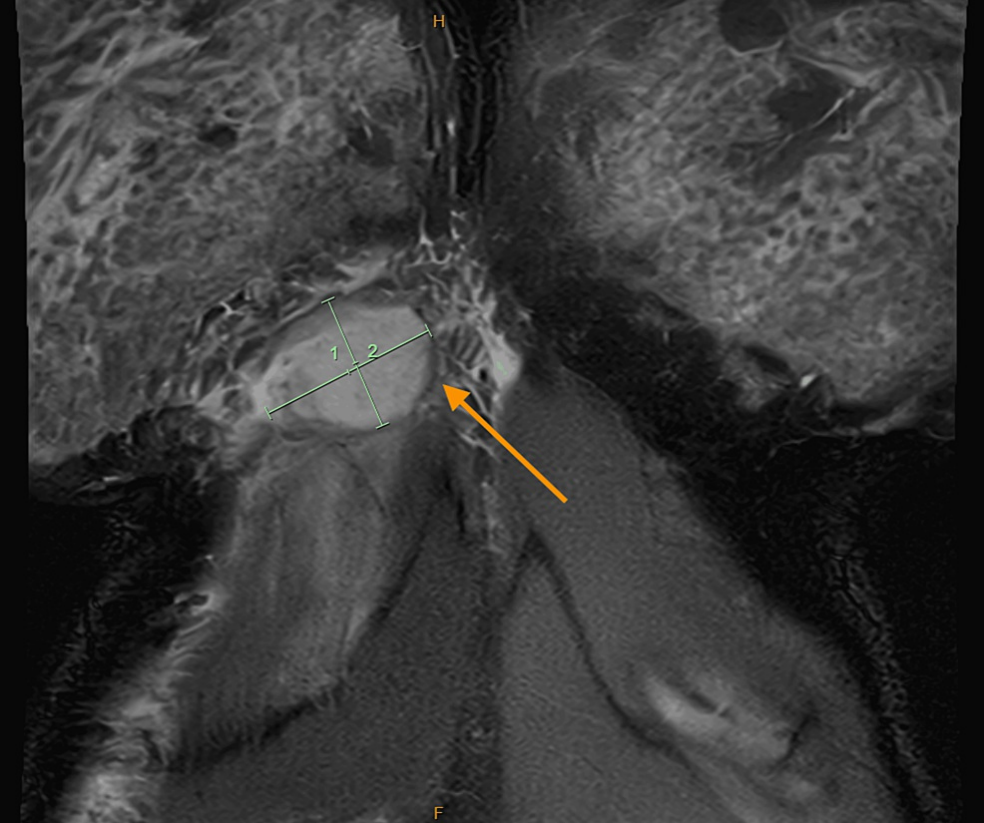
MRI chest wall (coronal). Inflammatory phlegmon/abscess at right chest wall overlying 6th costal cartilage. Length: 40.18mm (1) x 30.42mm (2)
